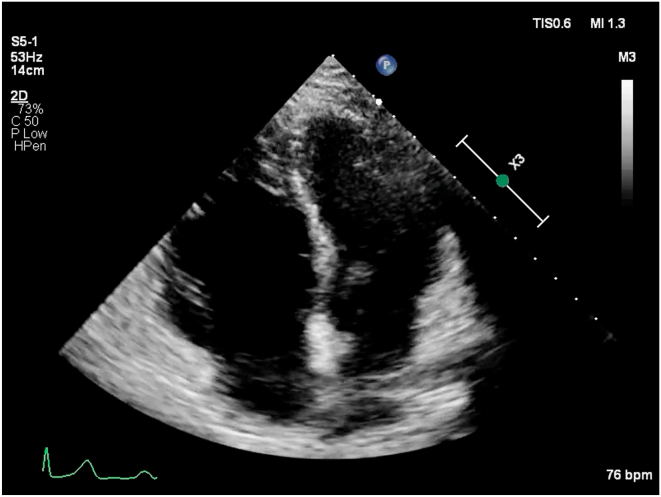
Two-dimensional TTE, apical 4-chamber diastolic view, demonstrates a dilated RV and RA with abnormal septal position consistent with right ventricular volume overload.
